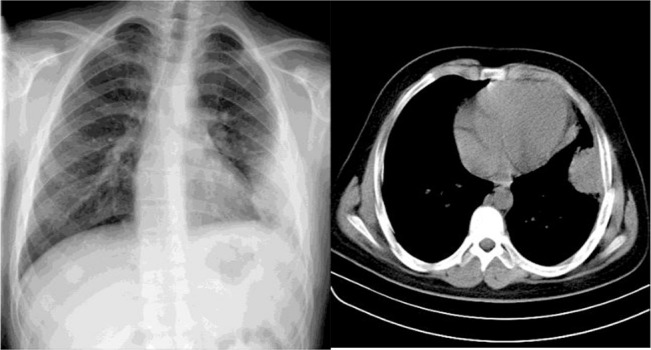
CXR shows a sub-pleural mass with silhouette sign. CT scan showed that there was a sub-pleural mass like consolidation in the LLL measuring up to 45×44mm associated with subtle ipsilateral pleural reaction. Right lung was intact.
